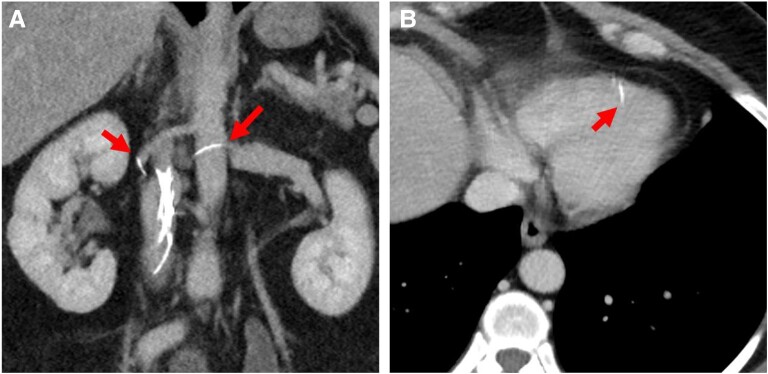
A 49-year-old patient was presented with compensated post-thrombotic syndrome in her left leg. In 2009, an inferior vena cava (IVC) filter was implanted due to iliofemoral deep vein thrombosis on the left side and bleeding while taking phenprocoumon as oral anticoagulation. To determine any thrombus burden in the area of the IVC filter, a CT angiography was performed at the MVZ Arabellahaus in Munich. This revealed a fractured IVC filter with penetration of the adjacent abdominal aorta and disseminated embolization of fragments into the left renal artery (*Panel A*), the pulmonary arteries, and the right ventricle (*Panel B*). The complication rate of a filter fracture is 1–2%, and the embolization of filter fragments is <1%. Penetration into neighbouring organs such as the intestine, aorta, or kidney occurs in 3.6% of cases and results in invasive therapy in 0.5%. In general, a cava filter that is no longer clinically indicated should be removed straightaway or implantation of temporary filters should be considered. Our patient was free of symptoms, so we refrained from any invasive therapy and planned clinical follow-ups.
